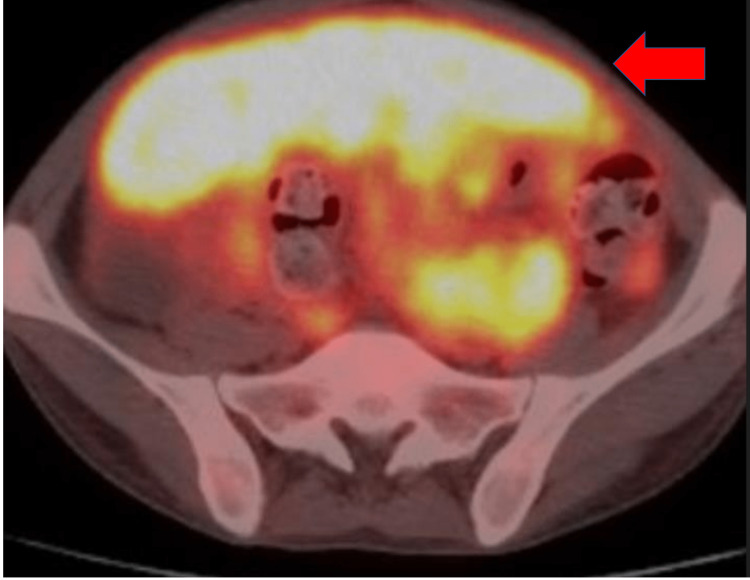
PET/CT demonstrating metastatic masses in the liver and abdomen along with mesenteric and peritoneal deposits (orange arrow) PET/CT: positron emission tomography and computed tomography
